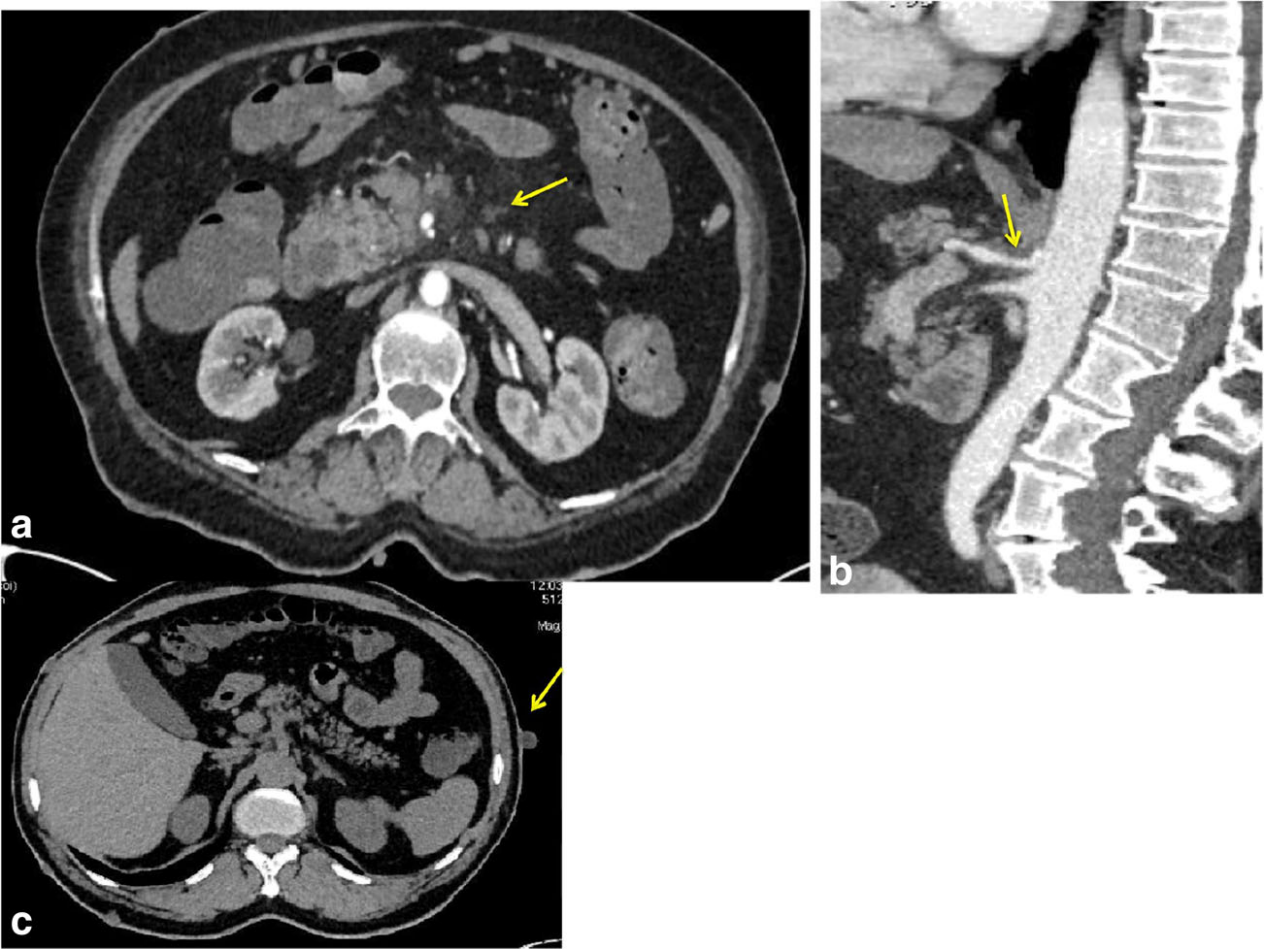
A 53-year-old male with a history of NF1. A small bowel CT scan showed no gastrointestinal tract involvement. a, b CT scan showed ostial stenosis of the coeliac trunk and superior mesenteric artery (arrows). c Multiple hypdense cutaneous nodules on both sides of the abdominal wall (neurofibromas)
